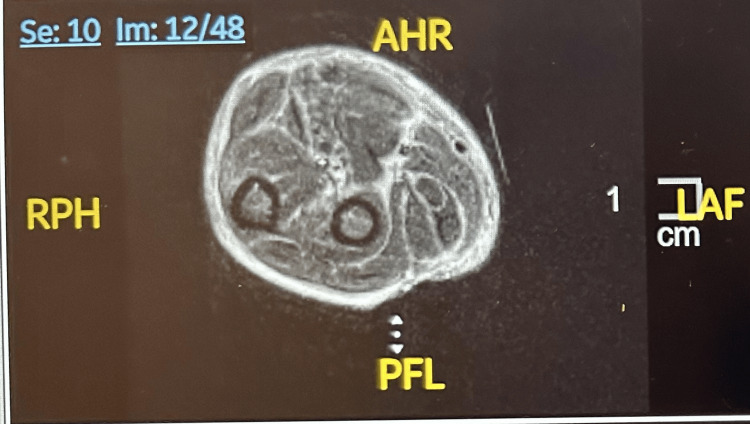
Axial MRI of the right forearm shows hyperintense signal along the deep fascial planes, consistent with diffuse fasciitis.
